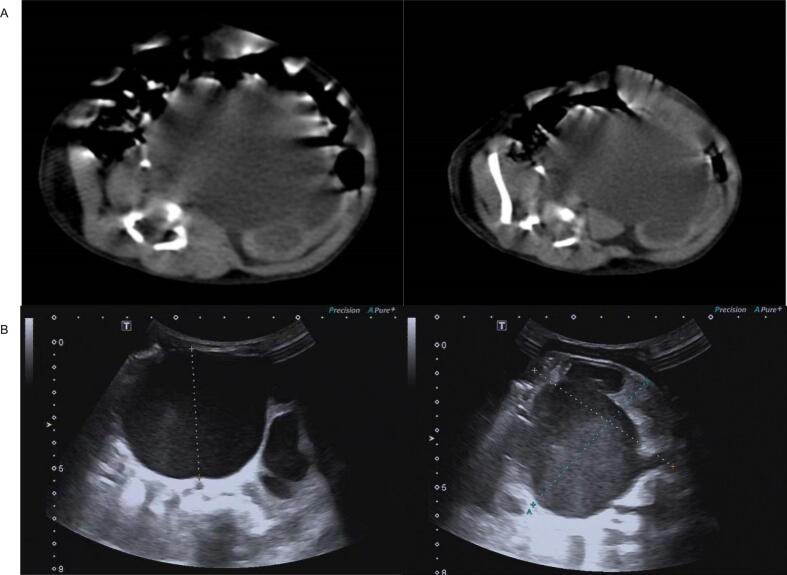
(A) Outcomes of preoperative CT indicated severe hydronephrosis and ureterectasis of left-sided moiety, along with left ureteral opening was dislocated; (B) Preoperative ultrasound examination indicated severe hydronephrosis.
